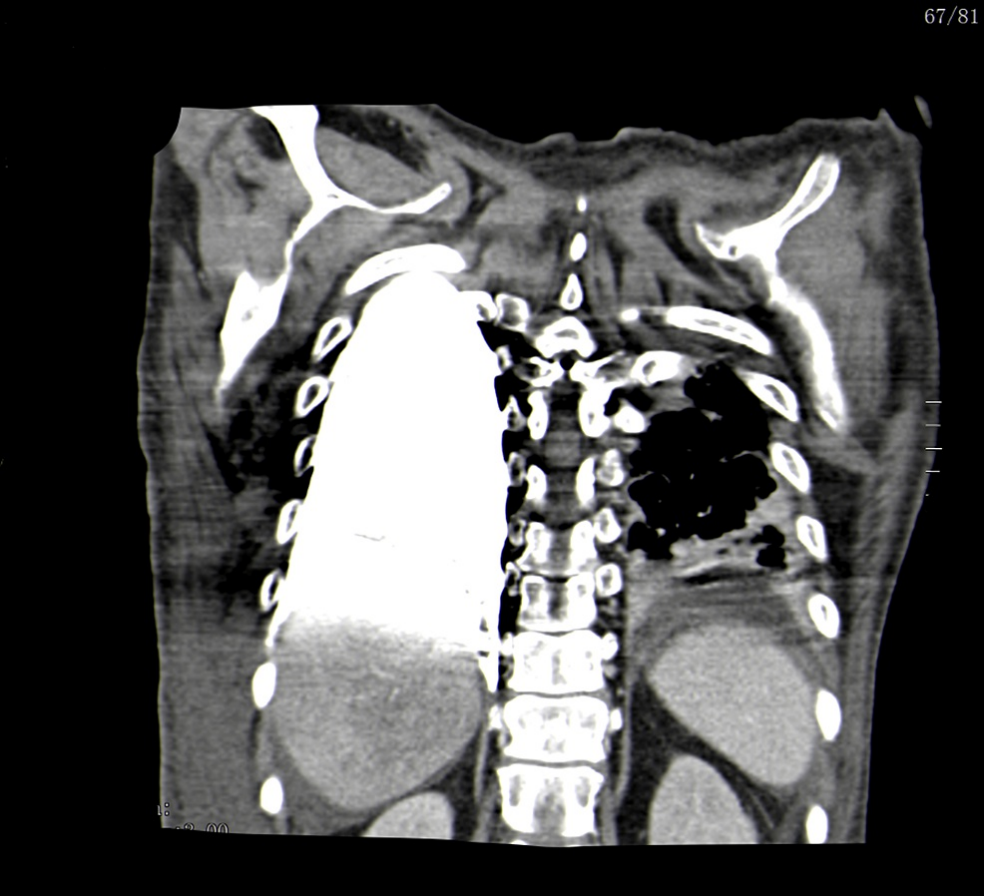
CTA scan showing contrast spreading through pleural space (coronal view) CTA, computed tomography angiography
